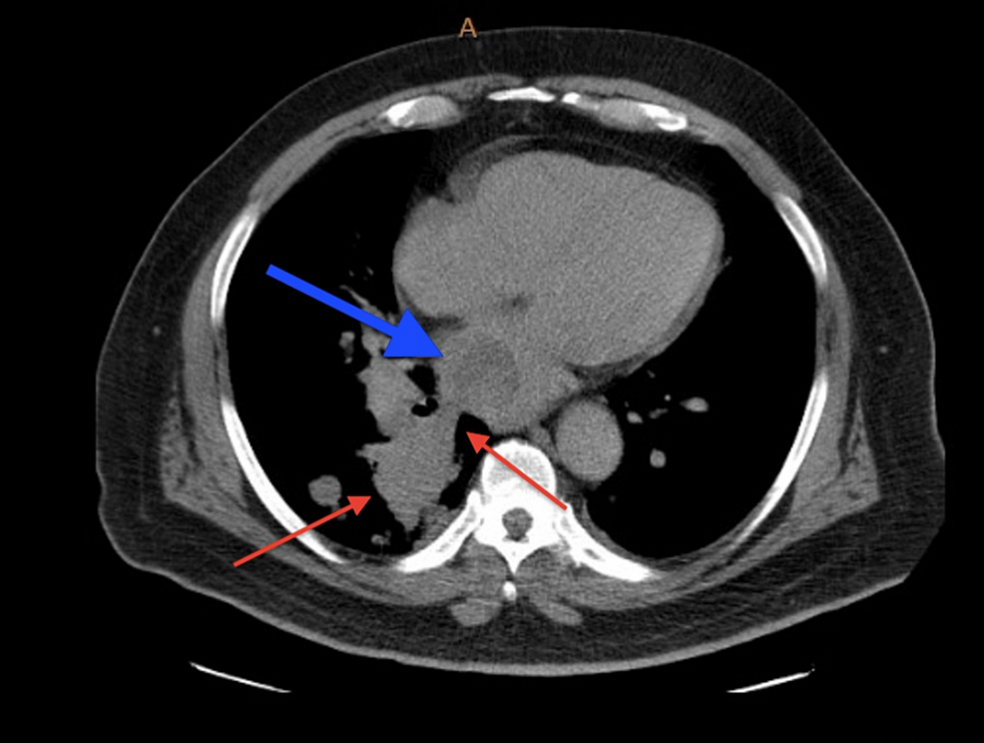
CT scan showing large right infrahilar mass consistent with malignancy (red arrows) with tumor invasion of the vasculature to the right lower lobe. Tumor invasion of the left atrium (blue arrow) of the heart versus left atrial thrombus.
